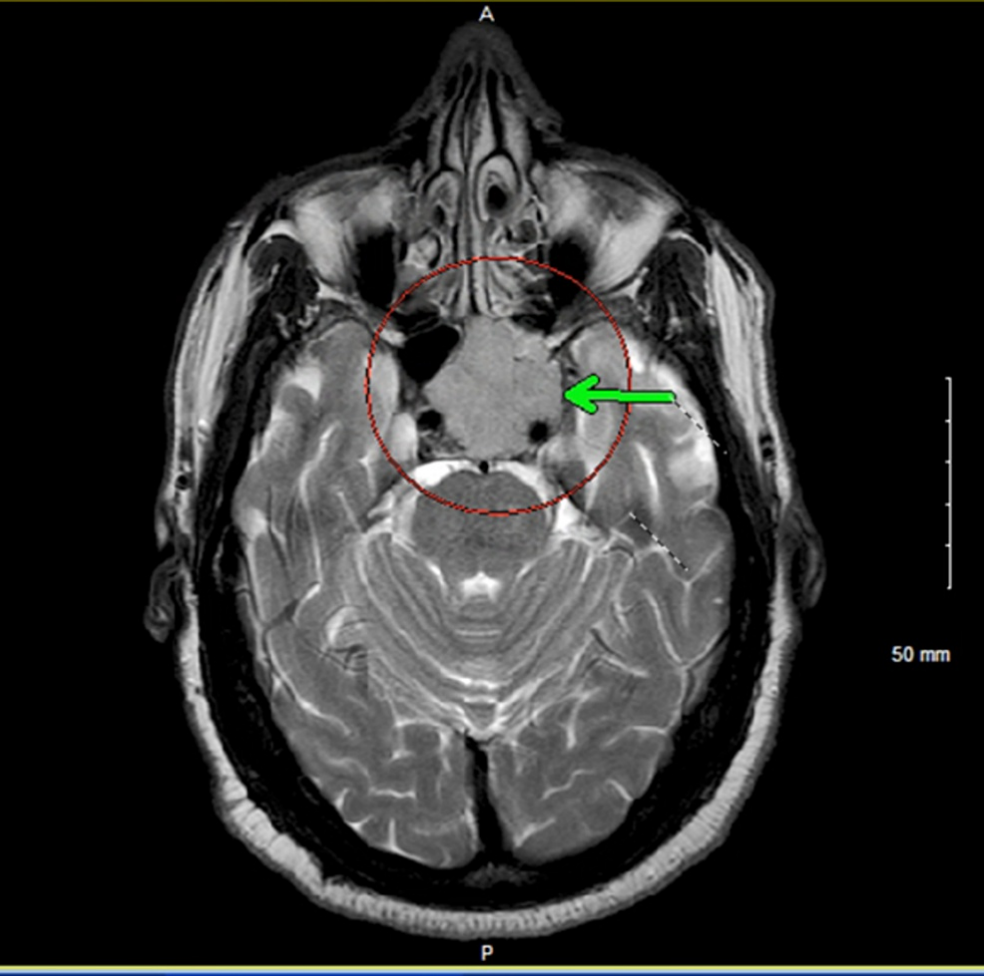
Axial T2-weighted MRI sequence showing the relationship of the mass with the optic nerve (green arrow). MRI: magnetic resonance imaging
